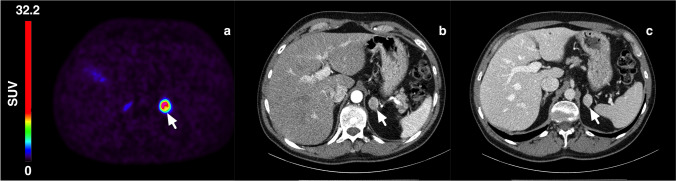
Left-sided adrenal Cushing(arrow), patient 3. [^18^F]CETO PET 3 h post injection (a), arterial phase contrast-enhanced CT (b), and venous phase contrast-enhanced CT (c), where CT was performed 3 days after [^18^F]CETO PET
